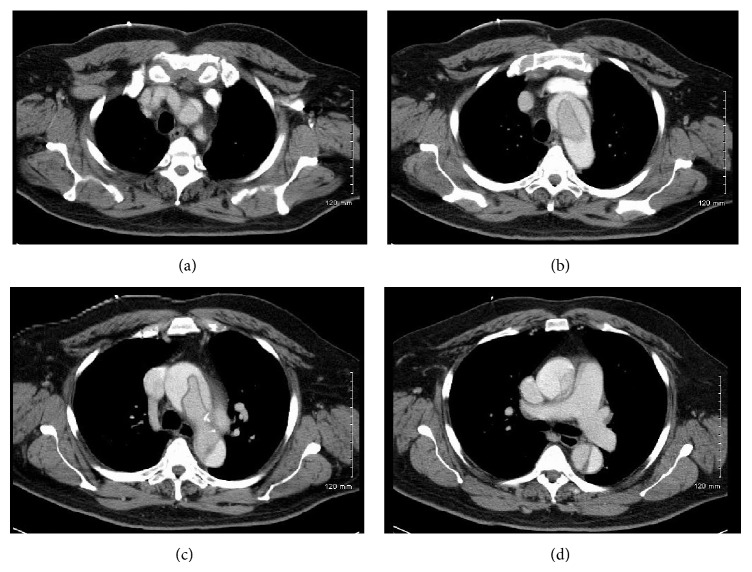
CT scan with contrast showing a thoracic aortic dissection involving the ascending aorta and descending aorta, with an intramural hematoma in the descending aorta. (a)–(d) show coronal sections at different levels.
